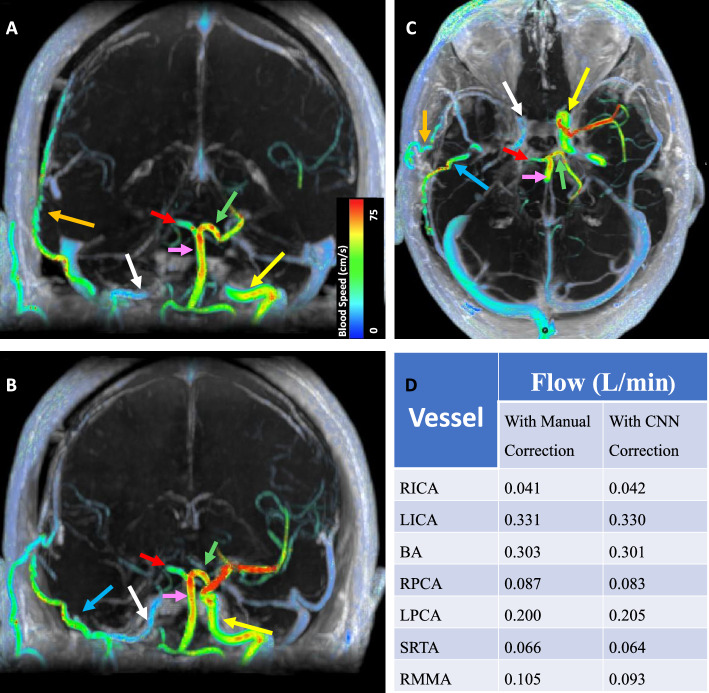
4D Flow MRI reveals sources of arterial inflow for the cerebrovascular circulation in a 35-year-old female patient with Moyomoya Disease (MMD) treated with right-sided extracranial-intracranial bypass. In both coronal and axial view (A–C), diminished right internal carotid artery (ICA) (white arrow) flow (0.041 L/min) is visualized. Left internal carotid artery (ICA) (yellow arrow) flow was 0.331 L/min. In both coronal and axial views (A–C), perfusion of the right middle cerebral artery territory from the right superficial temporal artery (STA) (orange arrow) bypass (0.066 L/min) and collateral flow from the right middle meningeal artery (0.105 L/min) (blue arrow) is visualized. The left middle cerebral artery is stenotic with high velocities in the MCA and asymmetric distribution of basilar artery (pink arrow) (0.303 L/min) flow to the left PCA with 0.200 L/min through the left PCA (green arrow) and only 0.087 L/min through the right PCA (red arrow). Flow measurements performed following CNN correction of background phase error did not differ significantly from flow measurements performed with manual correction (D). RICA, right internal carotid artery; LICA, left internal carotid artery; BA, basilar artery; RPCA, right posterior cerebral artery; LPCA, left posterior cerebral artery; RSTA, right superficial temporal artery; RMMA, right middle meningeal artery.
